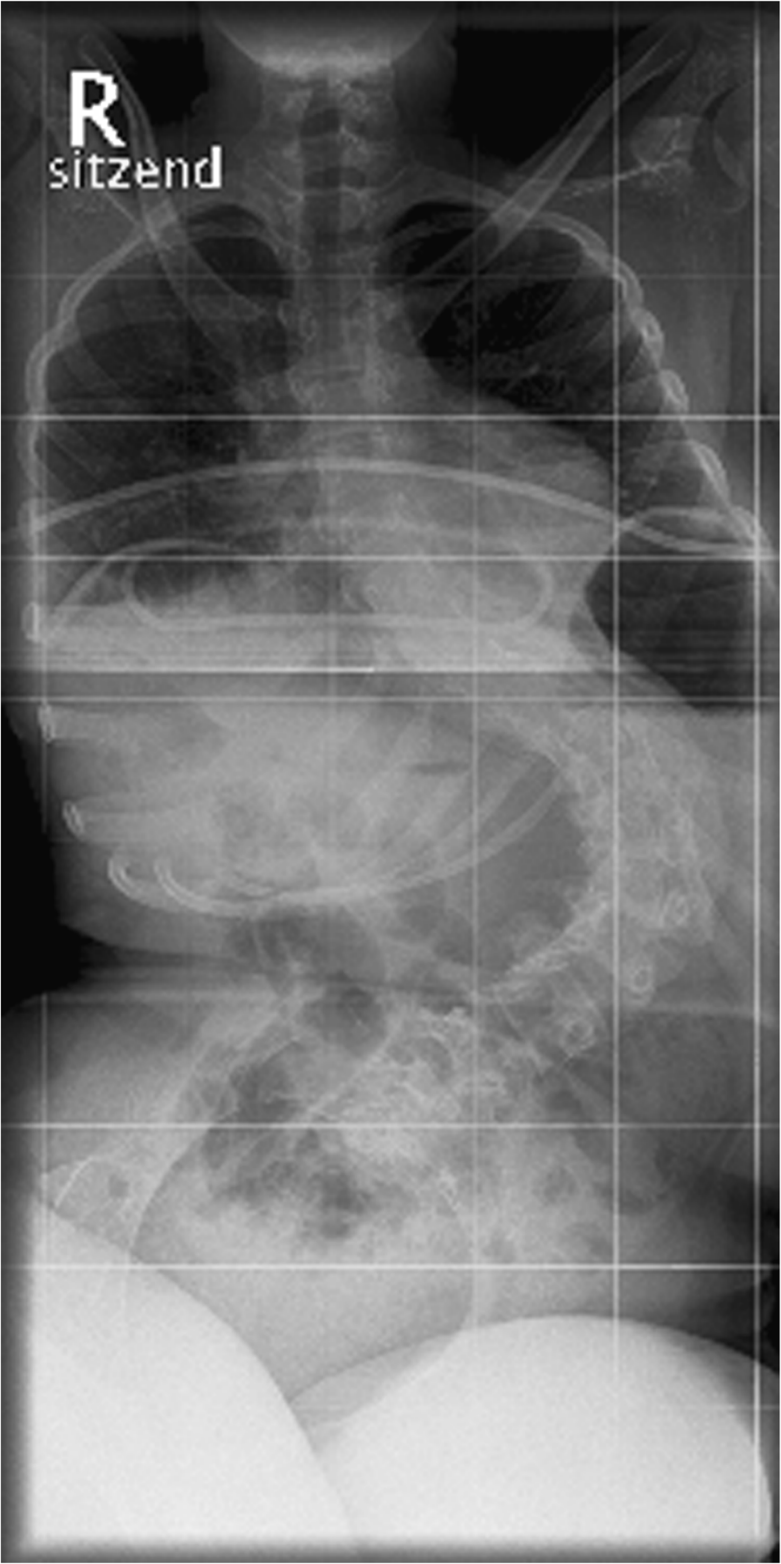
Anteroposterior preoperative X-ray of the spine: Cobb angle of 100° (Th8-L1-L5) left-convex, 45° pelvic tilt to the left.
